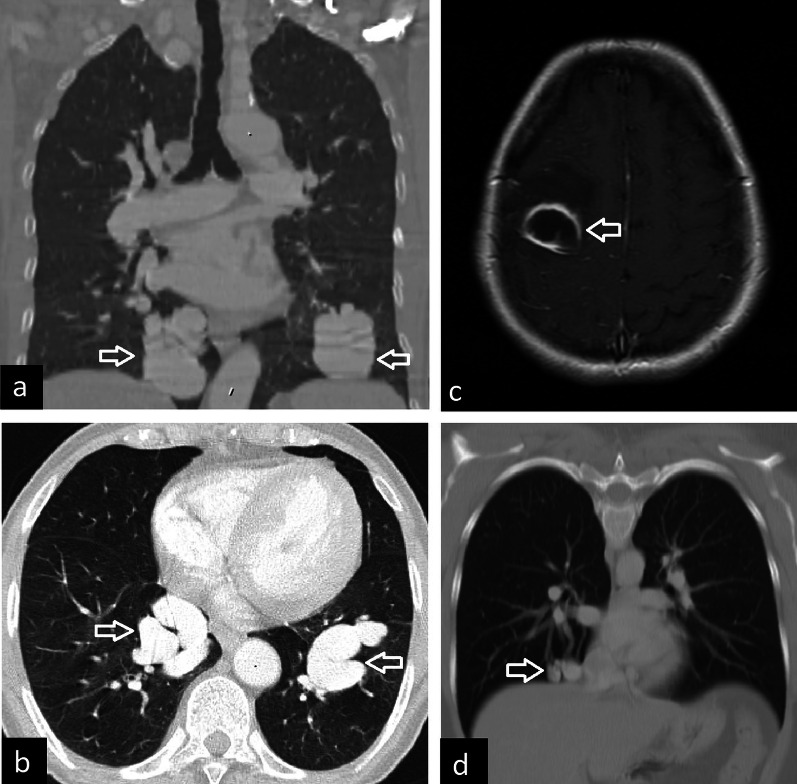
Pulmonary arteriovenous malformation (PAVM). Coronal (a) and axial (b) CT images show bilateral PAVM in a 69-year-old female (arrows). Contrast-enhanced T1-weighted MRI (c, arrow) depicts the brain abscess due to paradoxical embolization with ring enhancement and surrounding edema in a 36-year-old female. PAVM in the right lower lobe of the same patient is observed on coronal chest CT image (d, arrow)
